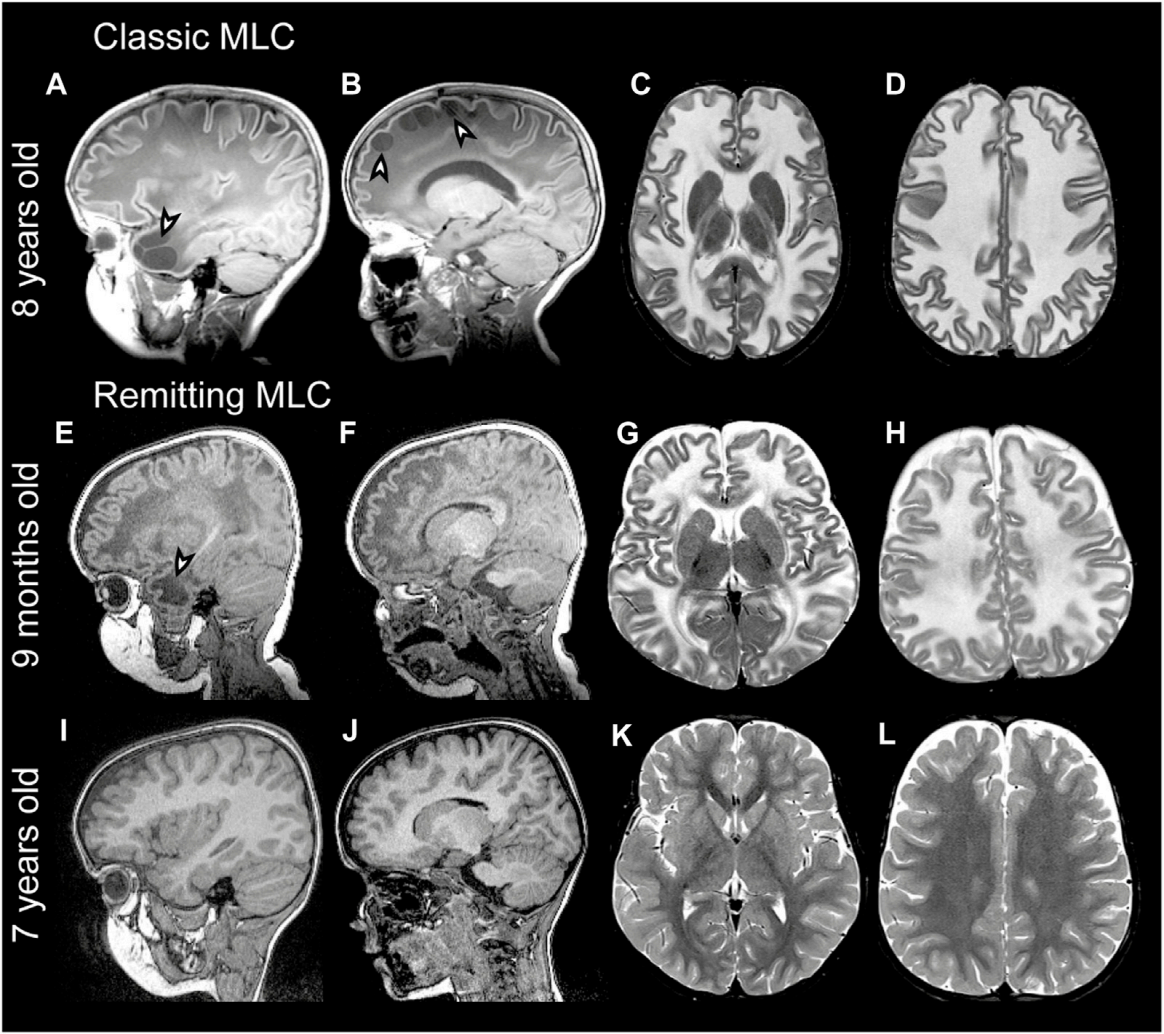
MRI findings in classic and remitting MLC patients. (A–D) MRI from an 11-year-old patient depicting an example of classic MLC. Anterior temporal and frontal subcortical cysts are visible in the sagittal T1-weighted MRIs in panels A and B (arrowheads). T2-weighted images in panel C and D show diffuse hyper intensity and swelling of the cerebral white matter with broadening of gyri (compare width of gyri in C and D to K and L). (E–H) MRI in a 9-month-old MLC patient and (I–L) images of the same patient at 7 years, showing the remitting phenotype. The anterior temporal cyst visible in panel E at 9 months (arrowhead) is no longer visible in panel I at 7 years. (F, J) No frontal subcortical cysts are present. Panels G, H, K, and L show that the cerebral white matter is initially T2-hyperintense and slightly swollen and that this T2-hyperintensity and swelling disappear over the years.
